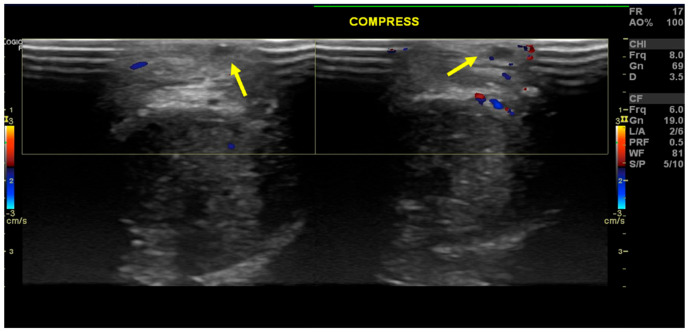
Axial Color-Doppler ultrasound of the penis. Arrows show the superficial dorsal vein. After compression of the penis, the vein remains non-compressible, another pathognomonic feature of this entity [1]. This case report is of great educational value, as many pathognomonic features of the disease are present on the ultrasound, namely the presence of endoluminal echogenicity, absent flow, and vessel incompressibility. Other characteristic findings include painful selective pressure, an increase in the caliber of the vein, and the presentation of inflammatory changes in the venous wall [1]. Differential diagnosis includes sclerosing lymphangitis and Peyronie’s disease, and is based on clinical history, physical examination, and ultrasound evaluation. Regarding ultrasonographic features, sclerosing lymphangitis presents with serpiginous (worm-like aspect) morphology, with thickened and dilated lymphatic vessels, without evidence of dorsal vein thrombosis. On the other hand, Peyronie’s disease presents as thickening of the tunica albuginea on the ultrasound, along with fibrotic plaques, which can be calcified [1].
